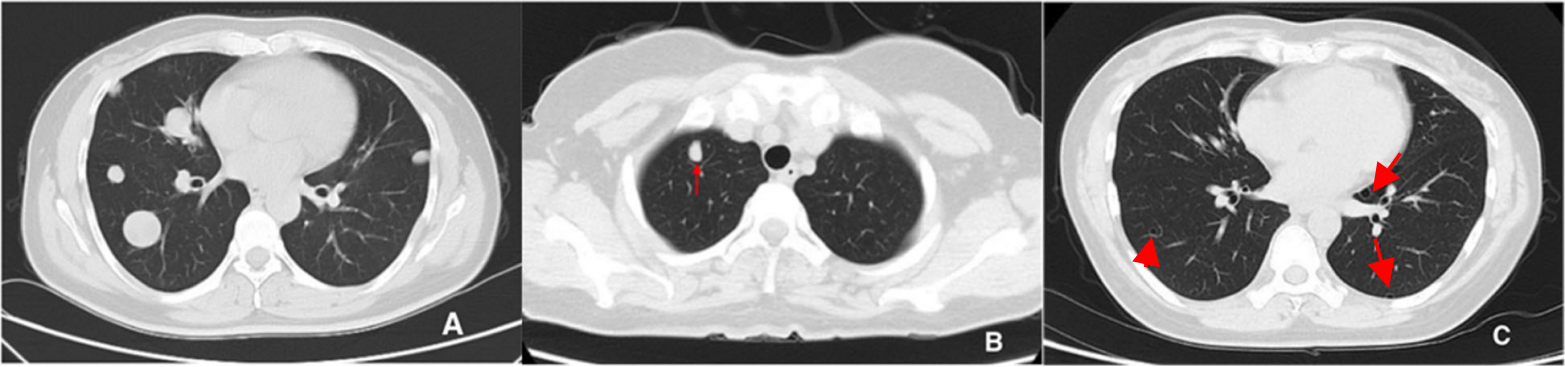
Chest computed tomography (CT) showed pulmonary benign metastasizing leiomyoma. a bilateral multiple nodules. b single nodule (arrow). c: bilateral multiple cysts (arrows)
